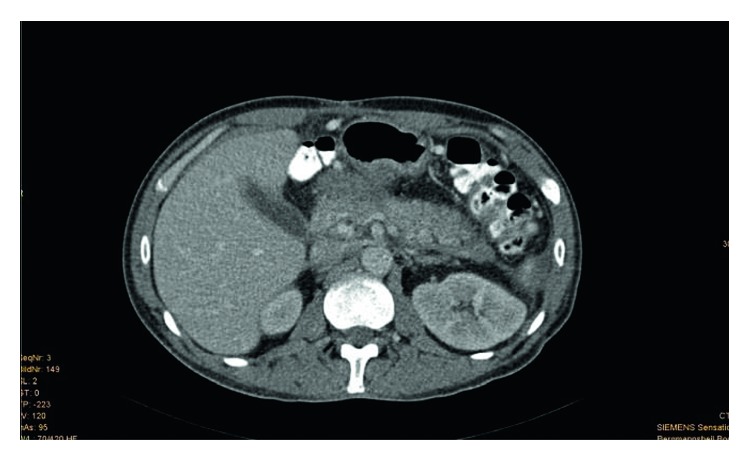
(Patient 1, scan 3) CT scan 4 months after trauma. Compared to the previous CT scan, no additional evidence of new pseudocyst or abscess formation was found. The drains that were previously placed in the bursa omentalis were removed.
